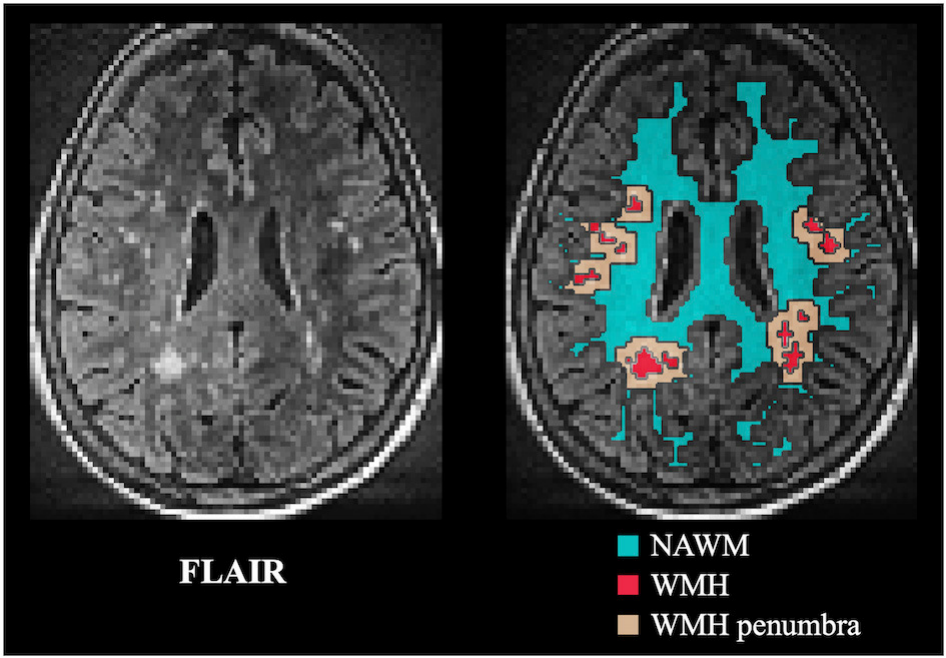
FLAIR image and overlaid template masks for NAWM, WMH and WMH penumbra (4-mm width). NAWM, normal appearing white matter; WMH, white matter hyperintensity.
